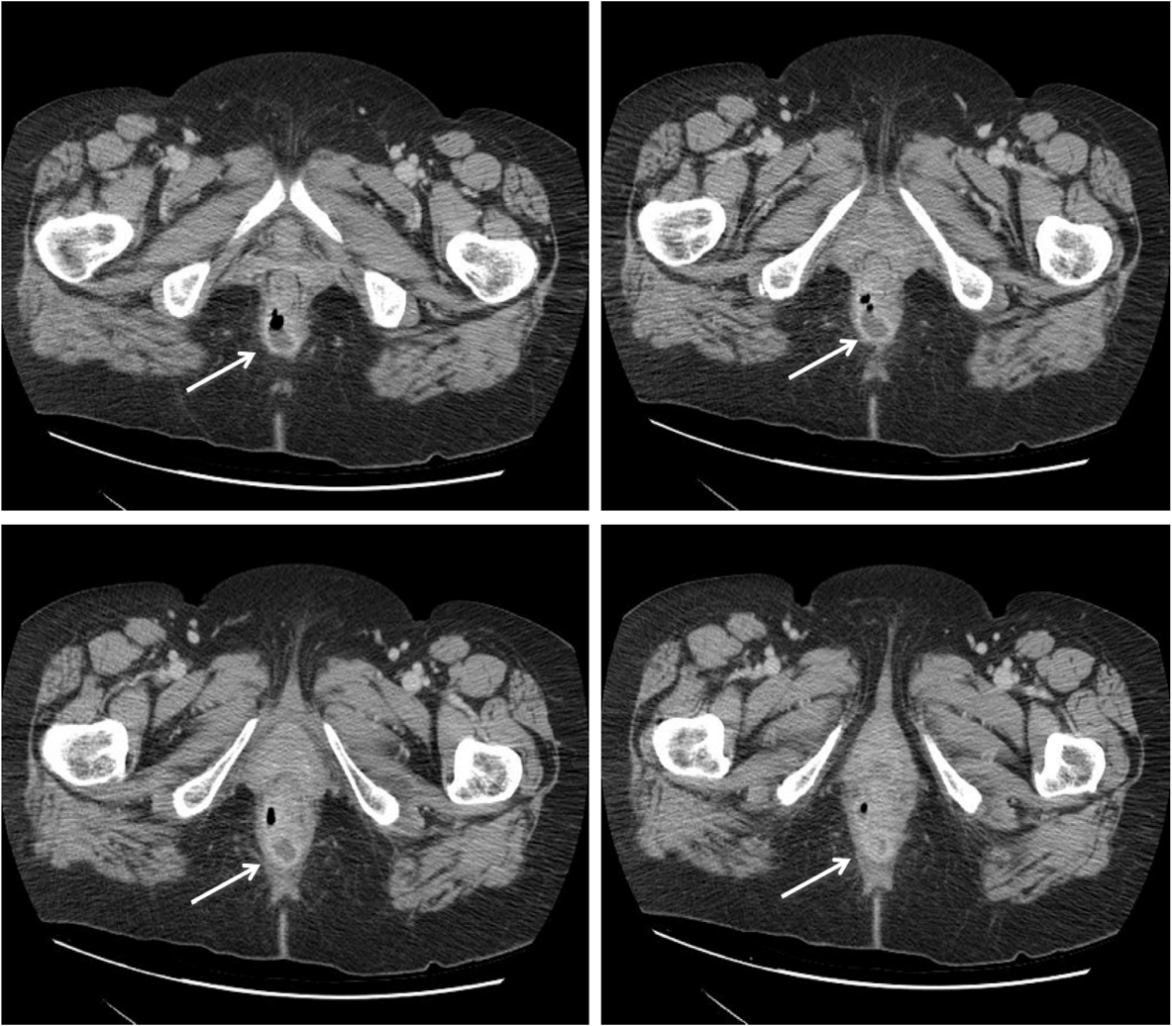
Abdominal CT scan. CT scan shows a small perianal lesion (32x30x28mm approximately) in the right posterior-lateral wall with hyperemic wall and partial gas content, probably an abscess (arrows). No free fluid in the pelvis
